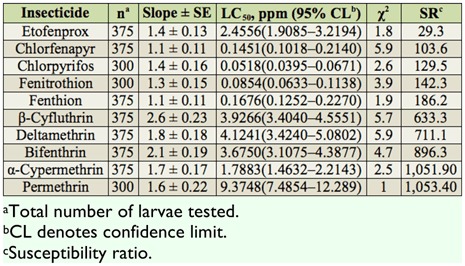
Summary of toxicities of 10 insecticides to third instars of the SS-CT strain of *Culex tritaeniorhynchus* based on the 24 hr exposure contact mortality bioassays.
